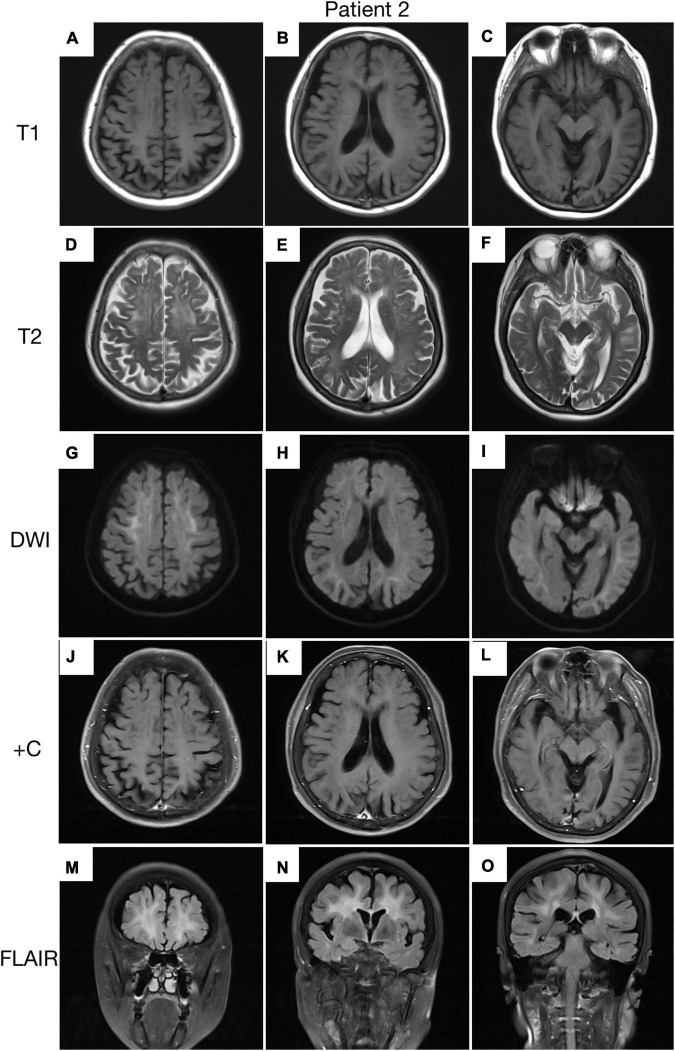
Brain MRI scan of patient 2. Cerebral atrophy was observed on T1 images (A–C). T2 (D–F) and FLAIR (M–O) images revealed severe leukoencephalopathy. Diffusion-weighted imaging (DWI) images revealed high linear signal in the corticomedullary junction (G–I). No significant enhancing lesions were observed (J–L).
